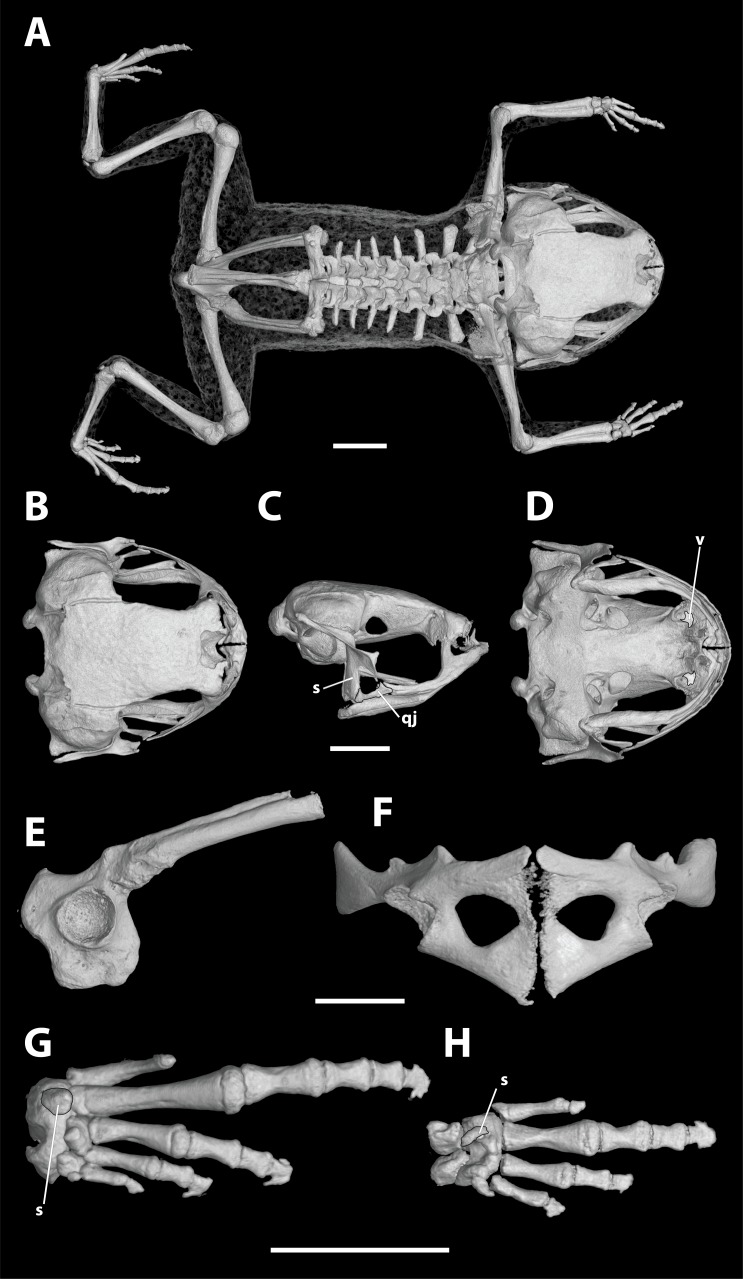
High-resolution computed tomography (CT) scans of a paratype of *Brachycephalus coloratus* (MHNCI 10274) showing key osteological features. While the vomer is fused to surrounding elements, we have highlighted its approximate boundaries. (A) Dorsal view of the skeleton; (B) dorsal, (C) lateral, and (D) ventral views of the skull (without the lower jaw); (E) ilium in lateral view; (F) pectoral girdle in ventral view; (G) right foot in plantar view; and (H) right hand in palmar view. Abbreviations: qj, quadratojugal; s, sesamoid; v, vomer. Scale bars equal 2 mm.
